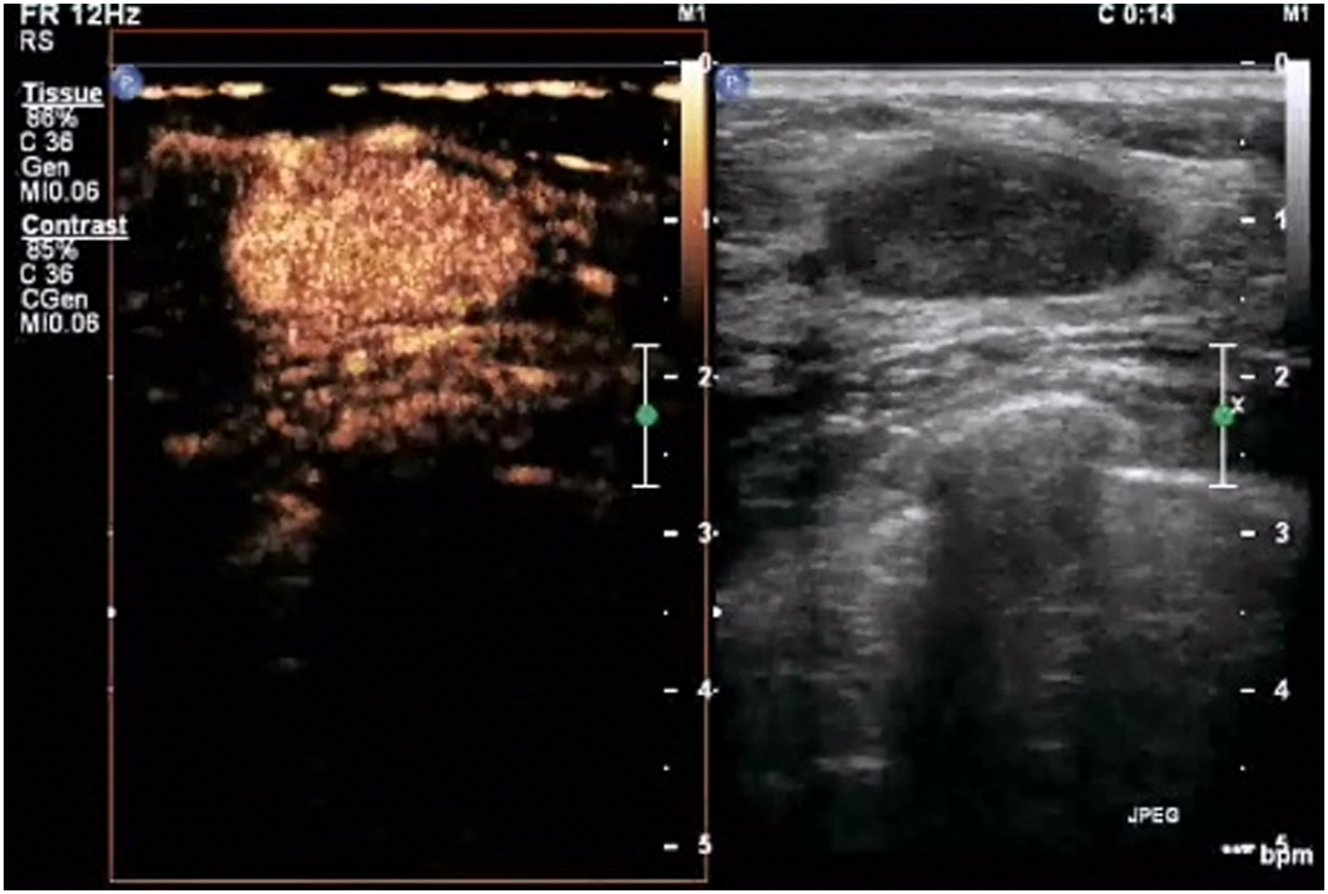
A lesion with a score of 3 by breast contrast-enhanced ultrasound. The lesion exhibits earlier enhancement compared with the surrounding tissue, homogeneous or heterogeneous, with a clear margin (sometimes with ring-like enhancement). The scope of the lesion is almost identical to that shown by 2D ultrasound.
